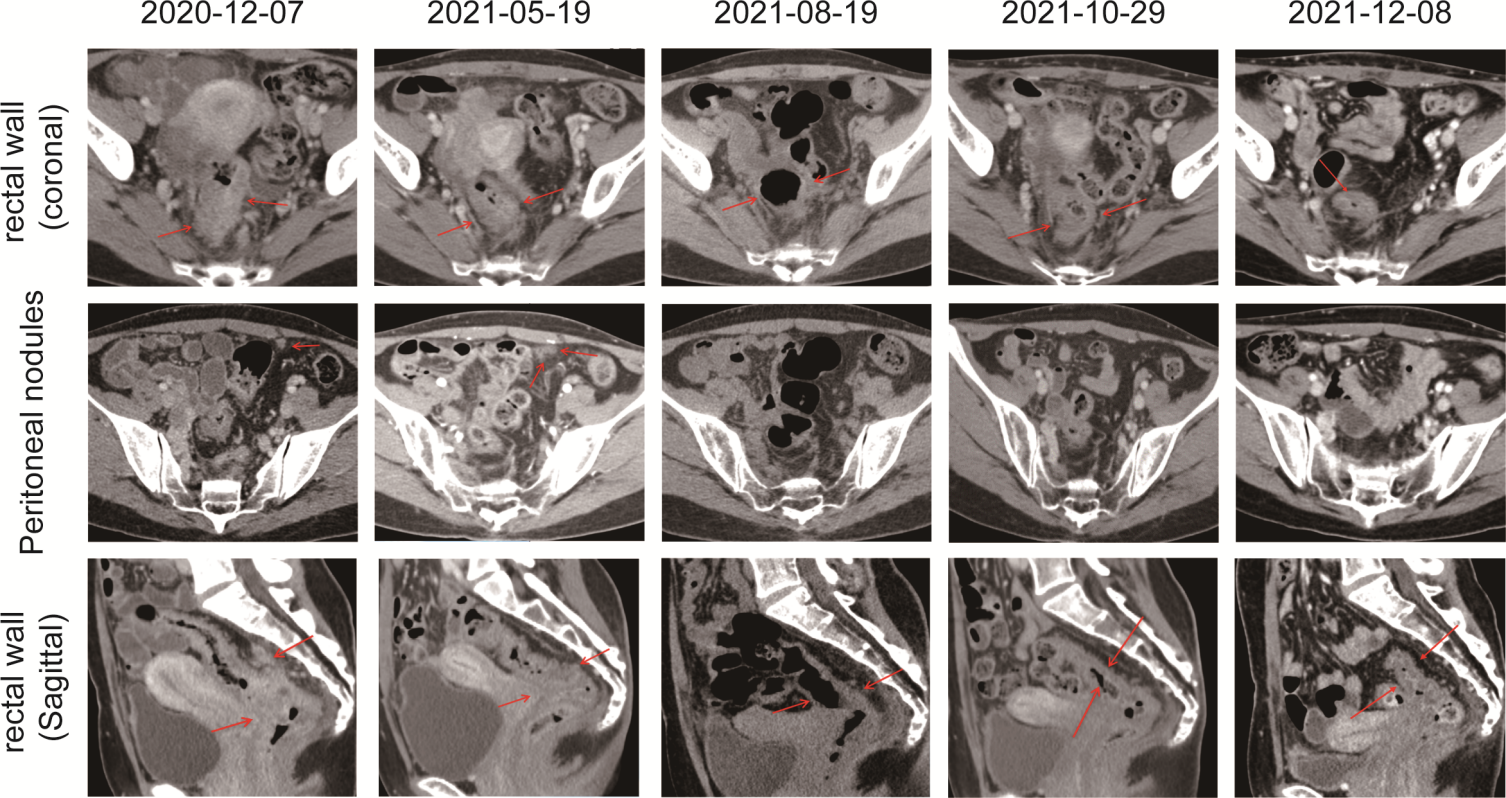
CT scans showed the chronological response of the patient following a series of treatment. The red arrow represents the area of the target lesion.
